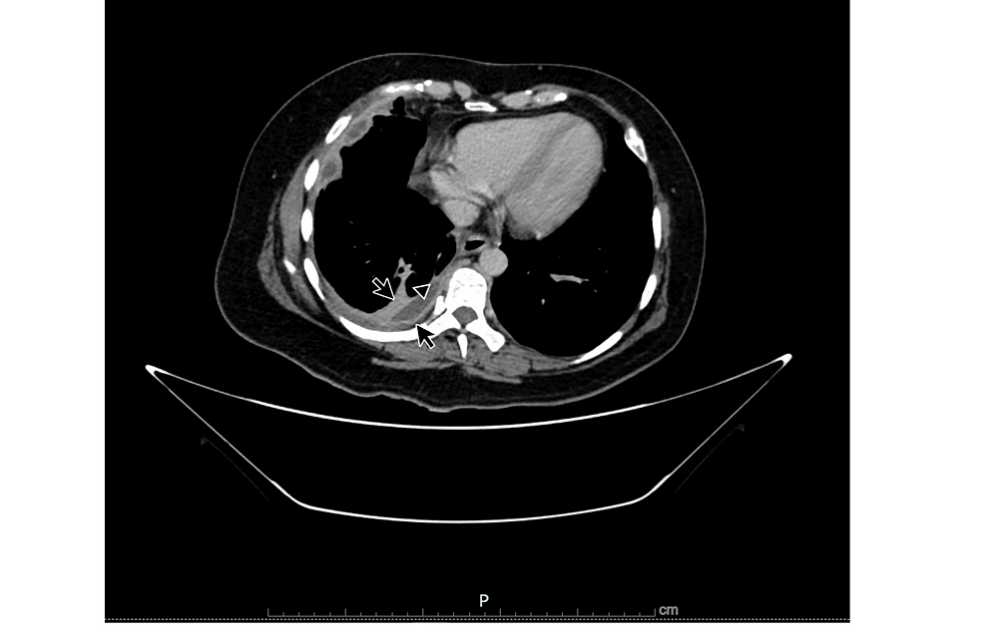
CT pulmonary axial view. Arrowhead: Heterogeneous hypodense material in the pleural space. Small arrows: Pleural thickening along with split sign.
